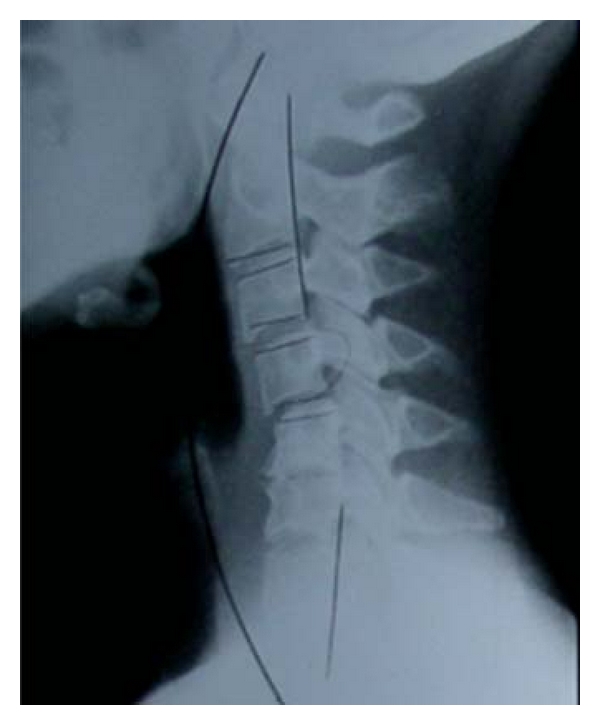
Simple radiograph demonstrating C4-C5 subluxation as well as C5-C6 degenerative spondylosis in a patient with symptoms of CSM.
